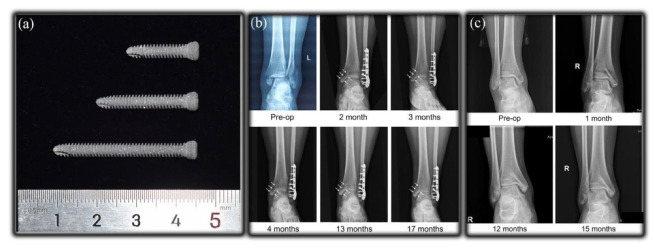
(a) The biodegradable Mg screw. (b) Preoperative and postoperative radiographs of a young female patient with a trimalleolar fracture. Two biodegradable JDBM screws (white arrows) are used to fix the medial malleolar fracture. (c) Preoperative and postoperative radiographs of a mid-age female patient with a medial malleolar fracture [217].
